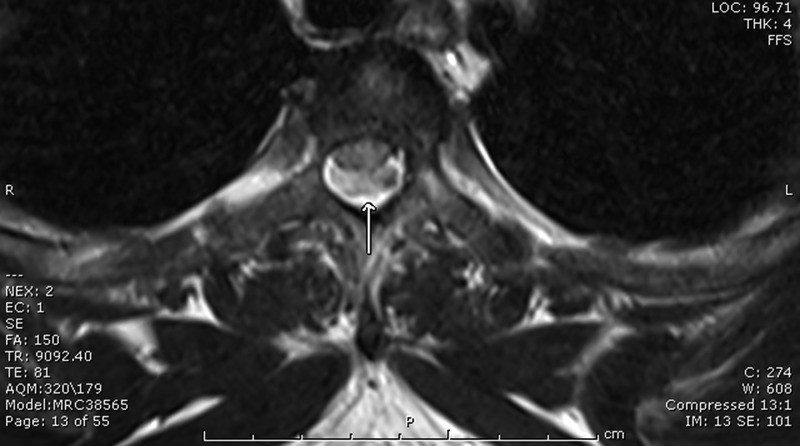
MRI of thoracic spine showed a mixed hyper- and hypo-intensity lesion on the T2 weighted image at the left posterior aspect of the thoracic thecal sac. The lesion compressed the thoracic spinal cord.
